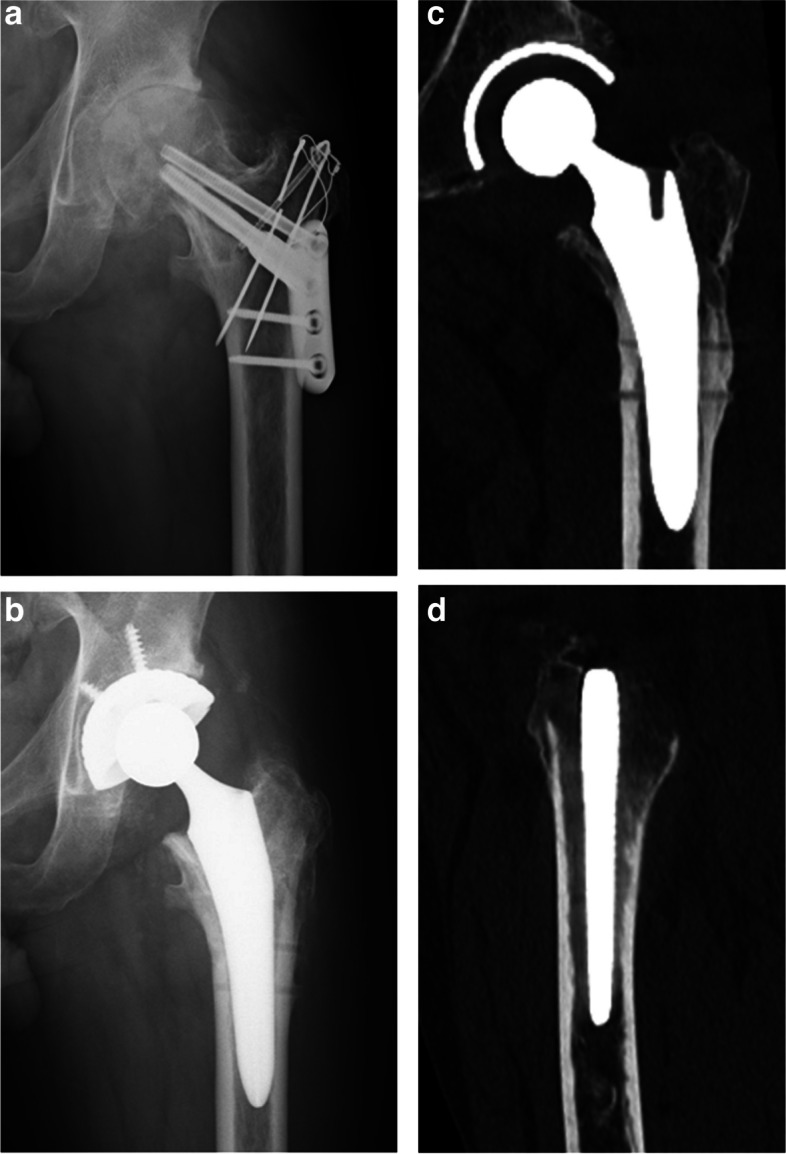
Fifty-four-year-old man with osteonecrosis of left femoral head related alcohol. a Radiological appearance of the left hip 11 years after transtrochanteric rotational osteotomy (TRO). b Radiological appearance after total hip arthroplasty (THA). c Postoperative CT coronal image showed 4° of varus alignment of the stem and osteosclerosis around the lag screw corridor in the medullary cavity. d Postoperative CT sagittal image showed 1° of extended alignment of the stem
